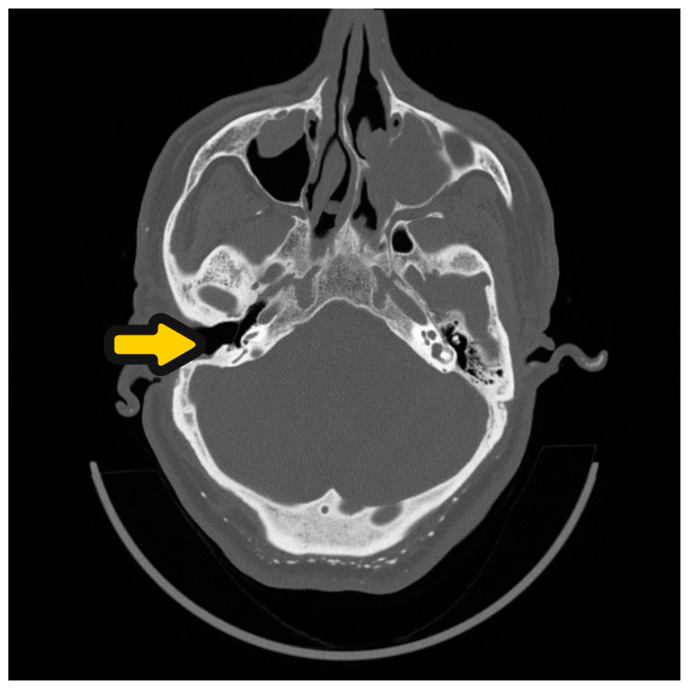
HRCT of the ear before SP and CI procedure. Open right-ear cavity after first ear surgery—yellow arrow.
